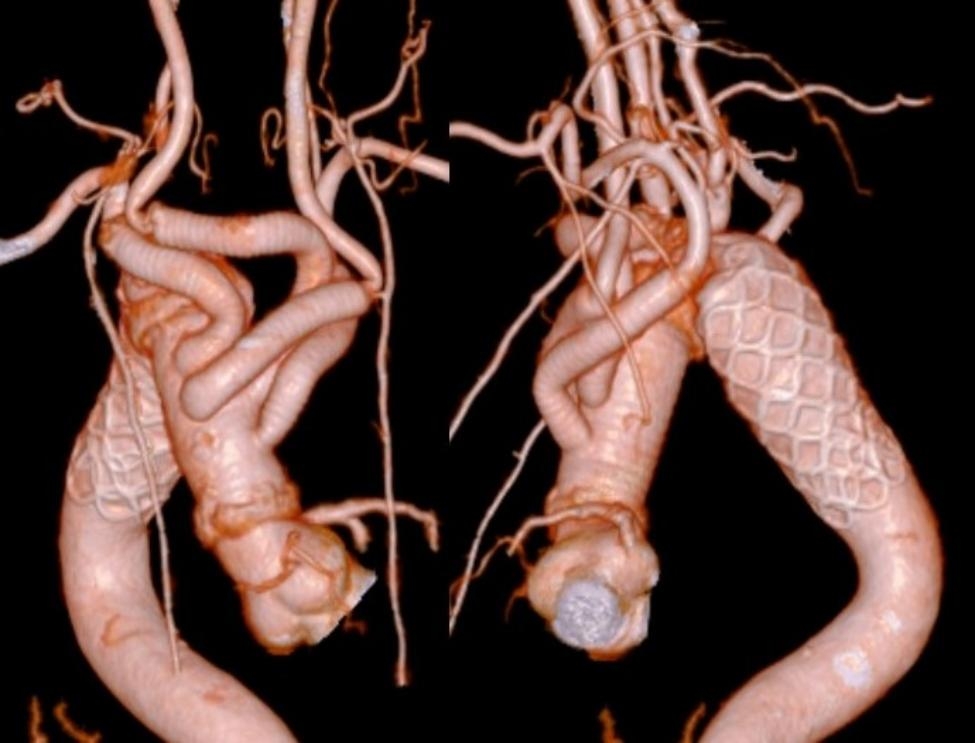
Follow-up computed tomographic angiography at 12 months postoperatively revealed no remaining Kommerell’s diverticulum with no endoleak
